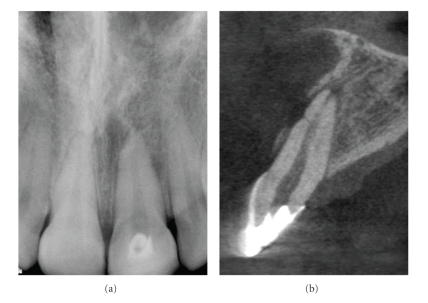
Traumatic injuries to the anterior dentition may result in a horizontal root fracture. Visualizing these fractures with periapical radiographs can be difficult as the beam must be in parallel alignment to the axis of the fracture. Conventional periapical image (a) shows horizontal radiolucent line separating the apical 1/3rd of the root. Note the loss of lamina dura and lateral radiolucency on the distal root surface adjacent to the horizontal radiolucency. Cross-sectional high-resolution (0.076 mm) image showing “V” shaped fracture and minimal displacement of the root segments. Note the loss of buccal cortical plate, widening of the buccal periodontal ligament space and periapical rarefaction.
